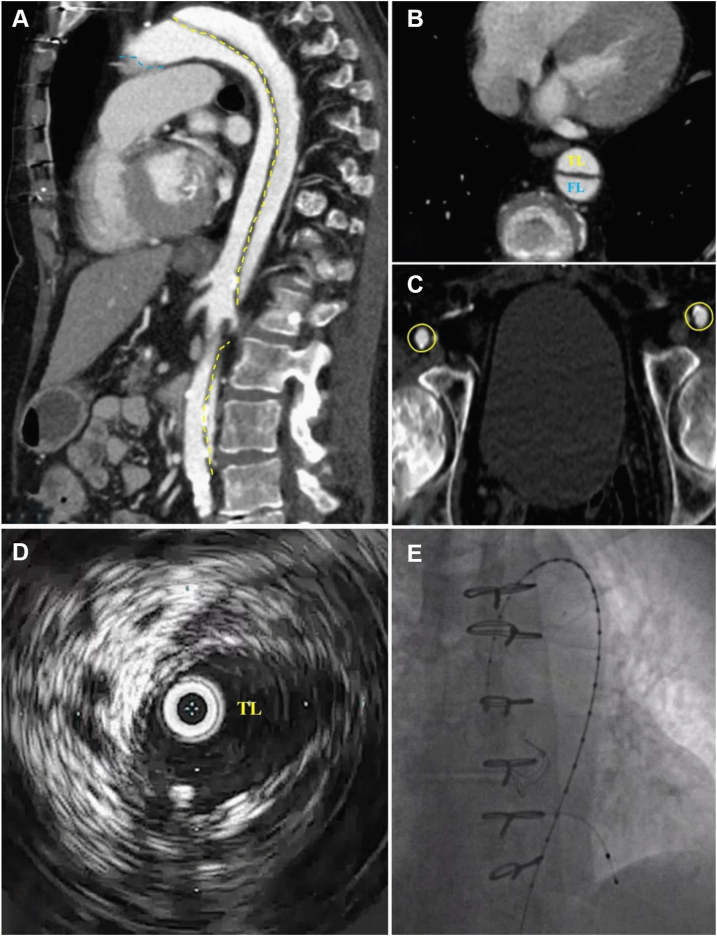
Preprocedural computed tomography assessment of chronic descending thoracic aortic dissection and intraoperative intravascular ultrasound–guided confirmation of true lumen access. (A) Curved planar reconstruction demonstrating the distal surgical anastomosis (blue dotted line) and a chronic descending thoracic aortic dissection with a noncircumferential intimal flap extending distally (yellow dotted line). (B) Axial computed tomography image of the descending thoracic aorta demonstrating preserved true lumen (TL) adjacent to the false lumen (FL). (C) Axial image at the level of the common femoral arteries demonstrating preserved, nondissected bilateral common femoral artery anatomy suitable for large-bore transfemoral access. (D) Intravascular ultrasound image demonstrating the catheter positioned within the TL during transfemoral access across a chronic descending thoracic aortic dissection. (E) Fluoroscopic image demonstrating intravascular ultrasound catheter advancement through the descending thoracic aorta. FL, false lumen; TL, true lumen.
